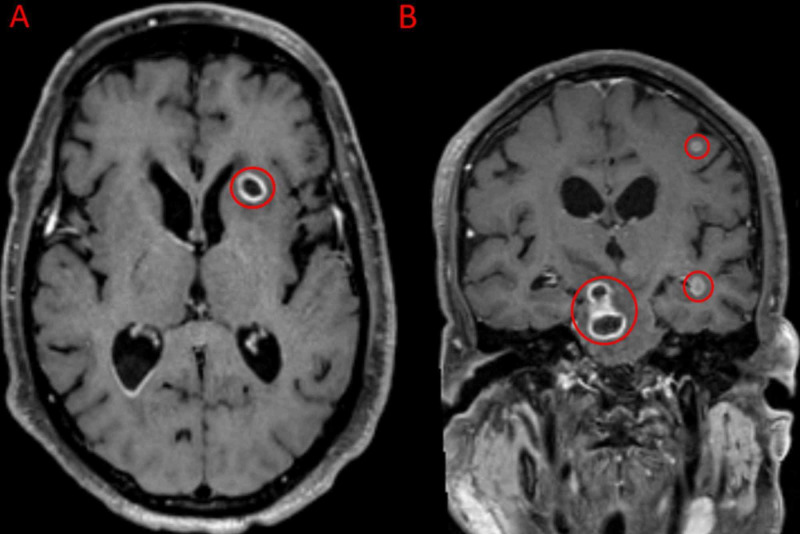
T1 Weighted MRI Demonstrating Concern for Abscesses or Septic Emboli T1 weighted MRI of the brain, post-contrast, in the axial (A) and coronal (B) planes demonstrate multifocal ring-enhancing lesions and hyperintense foci (red circles), concerning for subacute and acute abscesses or septic emboli. The most prominent lesion is located in right hemipons (B, largest red circle) measuring approximately 1.6 cm × 1.5 cm, with mild surrounding hyperintensity likely reflective of vasogenic edema.
